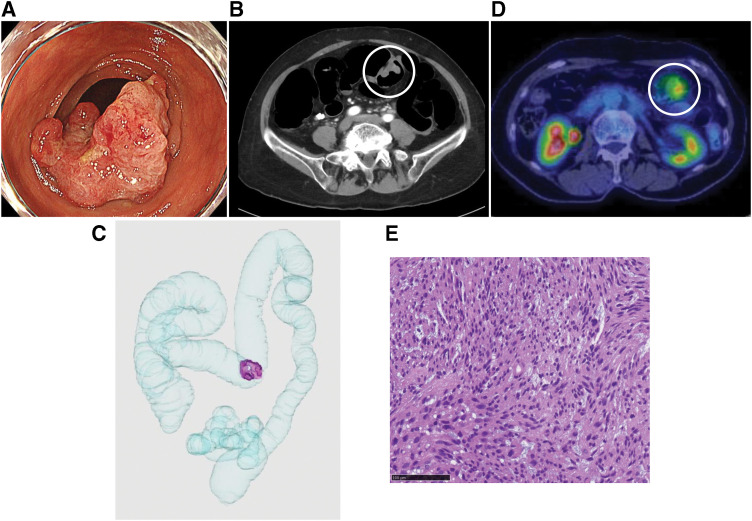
(A) Colonoscopy findings: A Bormann’s type 2 tumor was located in the transverse colon. (B, C) CT and CT colonography findings: CT and colonography revealed a 3-cm intradural mass in the middle transverse colon (circle and purple-colored mass). (D) PET-CT findings: PET-CT showed a mildly enhanced mass in the transverse colon (circle); there was no evidence of distant metastasis. (E) Pathological examination: The pathological finding revealed the spindle-shaped tumor cells (100 μm, scale bar). PET, positron emission tomography
